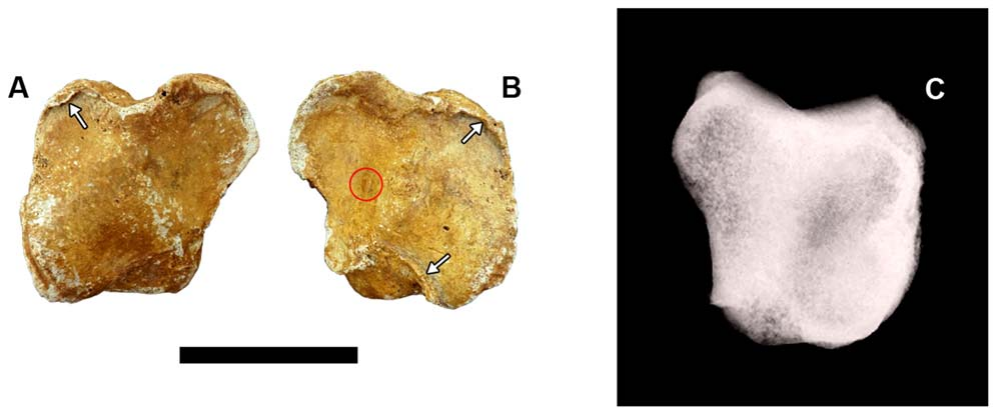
Patellae of *Glyptotherium*. (A) Right patella MCC 464-V in caudal view; (B) left patella MCC 473-V in caudal view; (C) Cranio-caudal radiography of the left patella. The white arrows indicate osteophytes, and the red circle indicates calcium deposition. Scale bar  =  5 cm.
